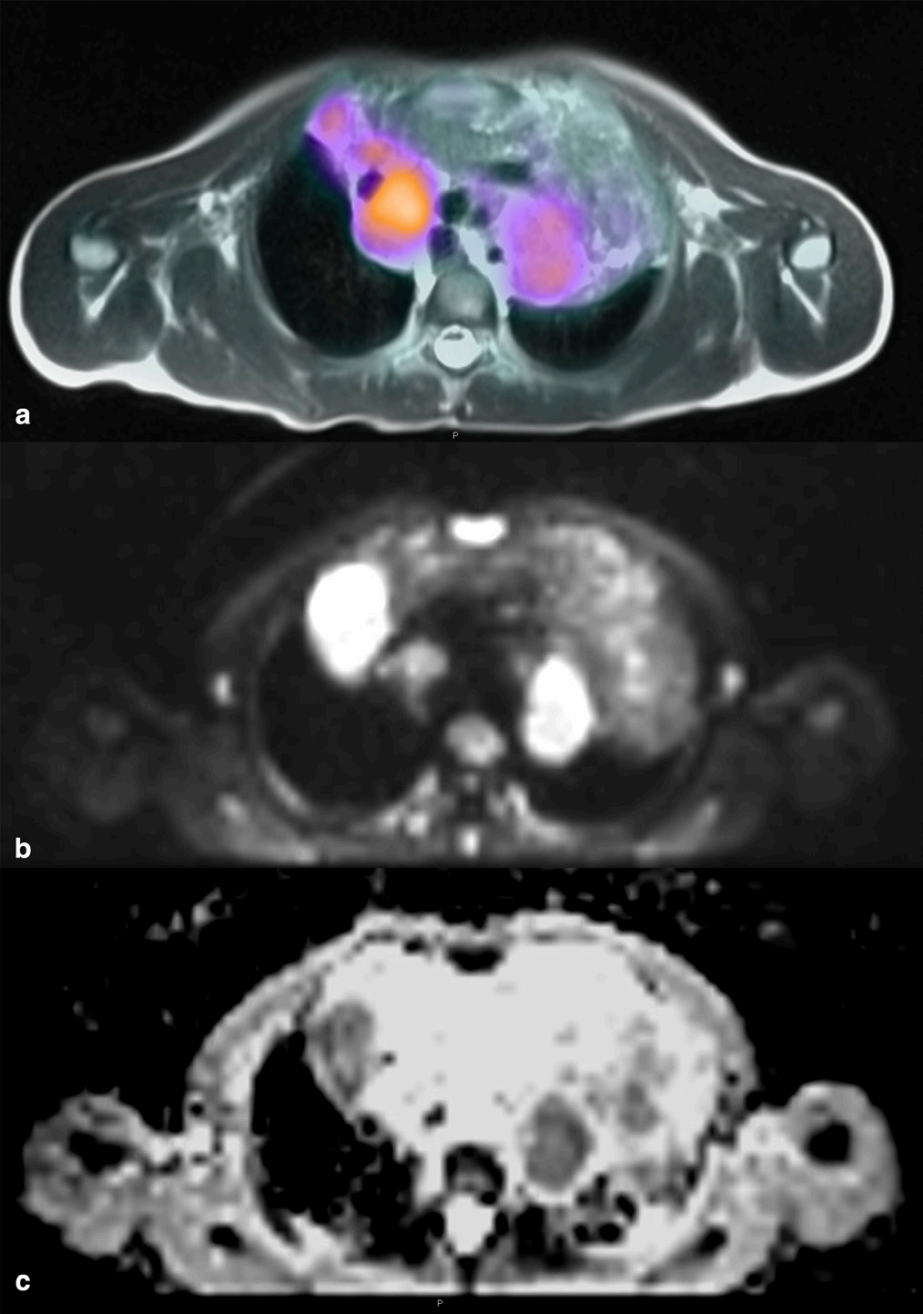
Axial fused 18-FDG-PET and T2 HASTE MRI examinations (A) demonstrating avid tracer uptake in a large mediastinal mass in a 9-year-old boy with Hodgkin’s Lymphoma. Axial diffusion weighted b800 image (B) and corresponding ADC map (C) demonstrating abnormal restricted diffusion in the same regions showing 18-FDG tracer avidity.
